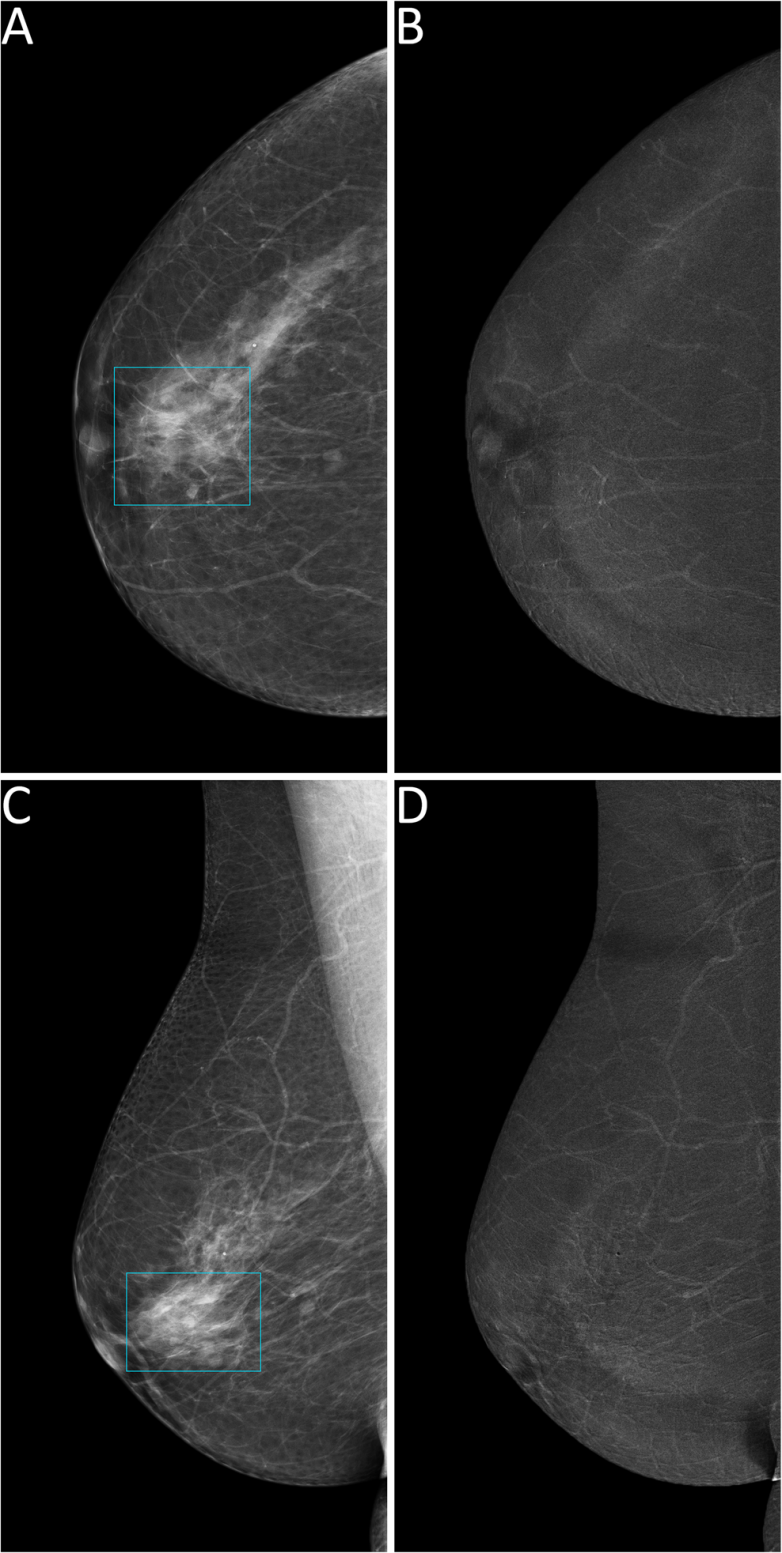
True negative case at contrast-enhanced mammography. A 58-year-old woman was recalled for a suspicious retroareolar irregular opacity in the right breast (panel A and C, light blue rectangles). An ultrasound-guided core needle biopsy was performed, leading to a diagnosis of apocrine metaplasia. The absence of enhancement foci on recombined images (panels B and D) would have oriented the referral to follow-up
